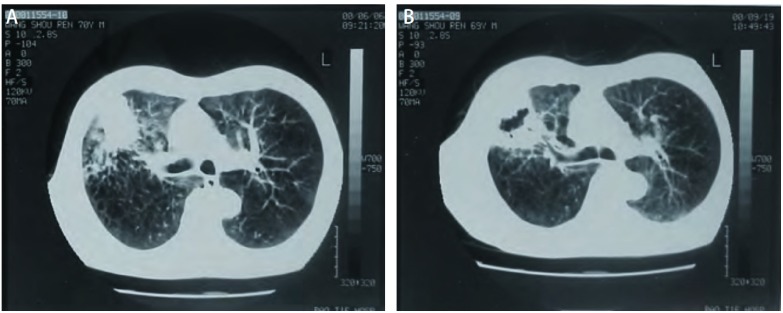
70岁老年男性患有右肺腺癌。A：术前CT影像显示可见右肺上叶4.2 cm大小肿瘤; B：术后3月可见右肺上叶空洞样改变。 70-year man with primary lung adenocarcinoma. A: CT image shows a lung tumor measuring 4.2 cm in the right upper lung before RFA. B: CT image shows a cavity in the right upper lung at 3 months after RFA. RFA: radiofrequency ablation.
